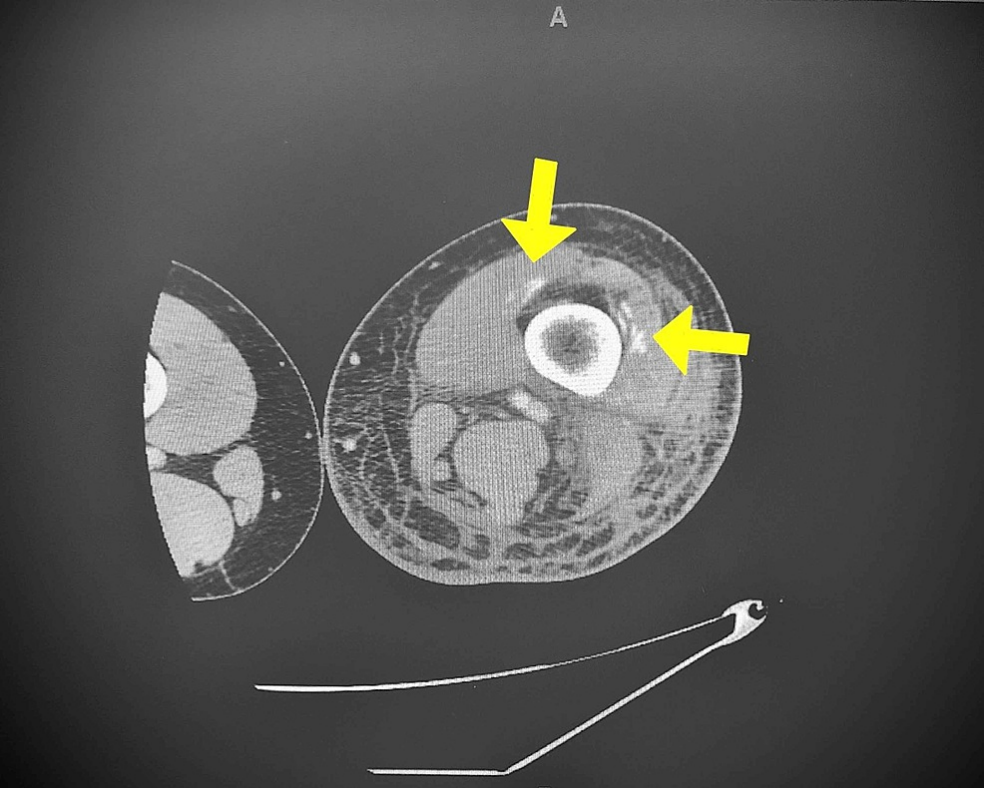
CT (axial view) of the left lower extremity. Curvilinear calcifications within the distal vastus intermedius, vastus lateralis, and vastus medialis.
